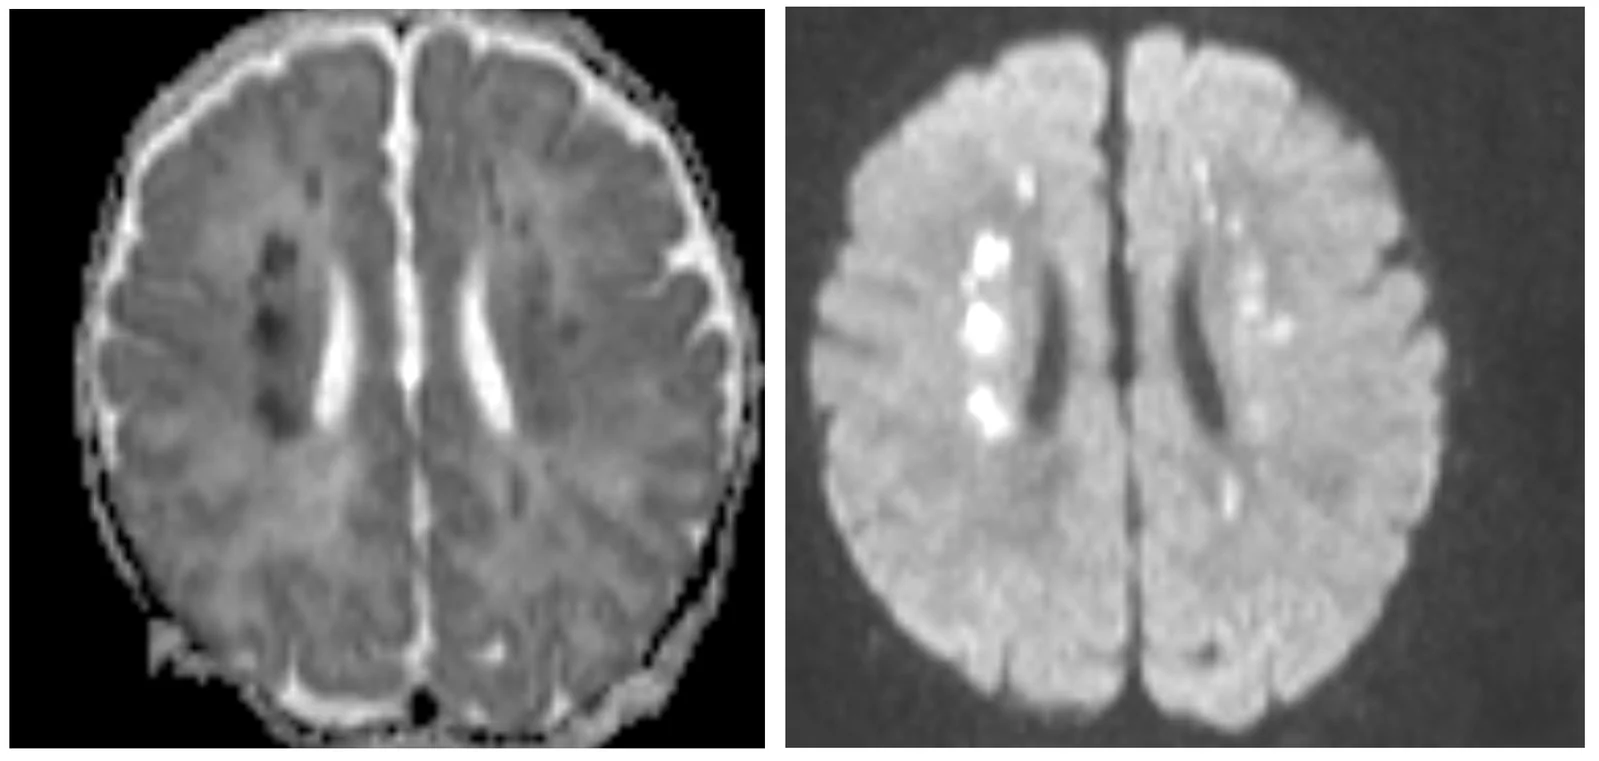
Images demonstrating restricted diffusion in the distribution of the watershed zone as a result of ischemic injury, with hyperintensity in the diffusion-weighted image (DWI, (left)) and corresponding hypointensity in the apparent diffusion coefficient (ADC, (right)). Images are original and obtained from Rady Children’s Hospital’s deidentified patient cases.
